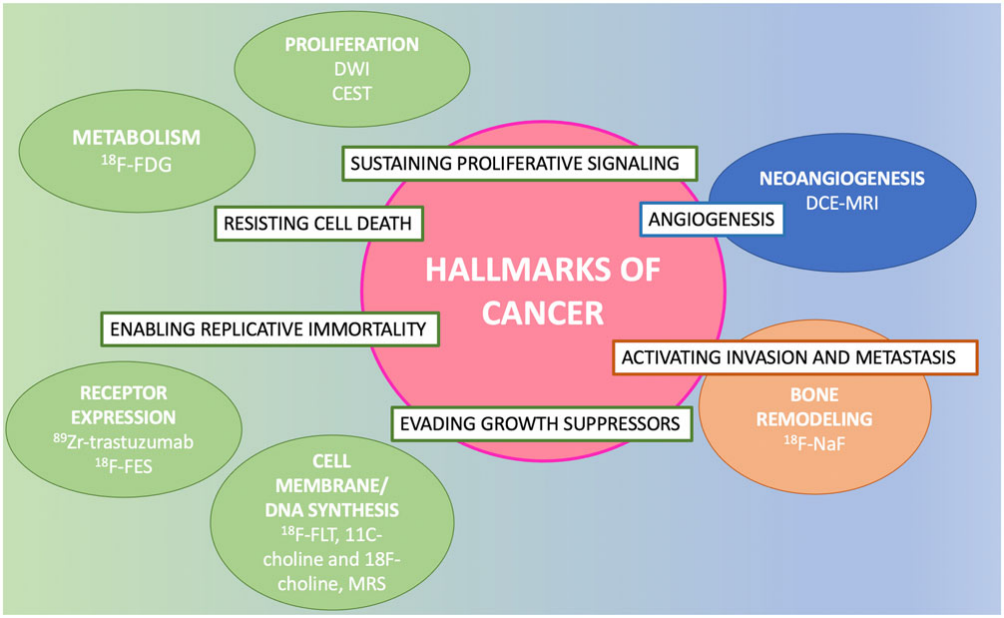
Hallmarks of cancer with corresponding PET and MRI biomarkers.
